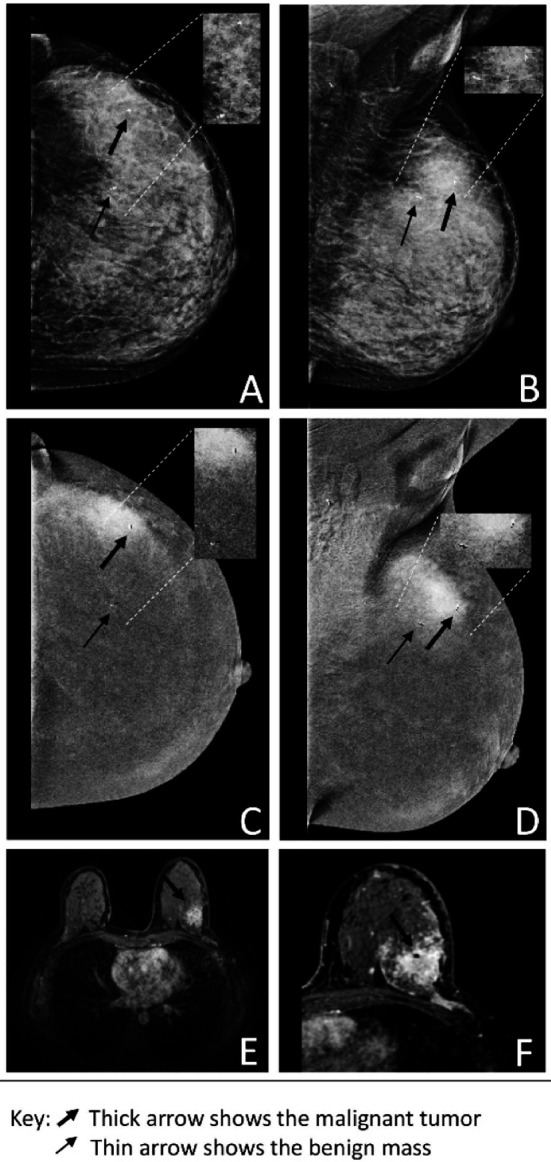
Low energy imaging CC and MLO view of the left breast (A & B). Recombined image CC and MLO view of the left breast (C & D) demonstrating biopsy-proven malignant lesion with a clip at 2 o’clock (upper outer quadrant) showing enhancement and a biopsy-proven benign lesion at 12 o’clock with the clip showing no enhancement. MRI of the left breast (E & F) shows an area of enhancement in the upper outer quadrant with a signal void area along with a post-biopsy clip.
